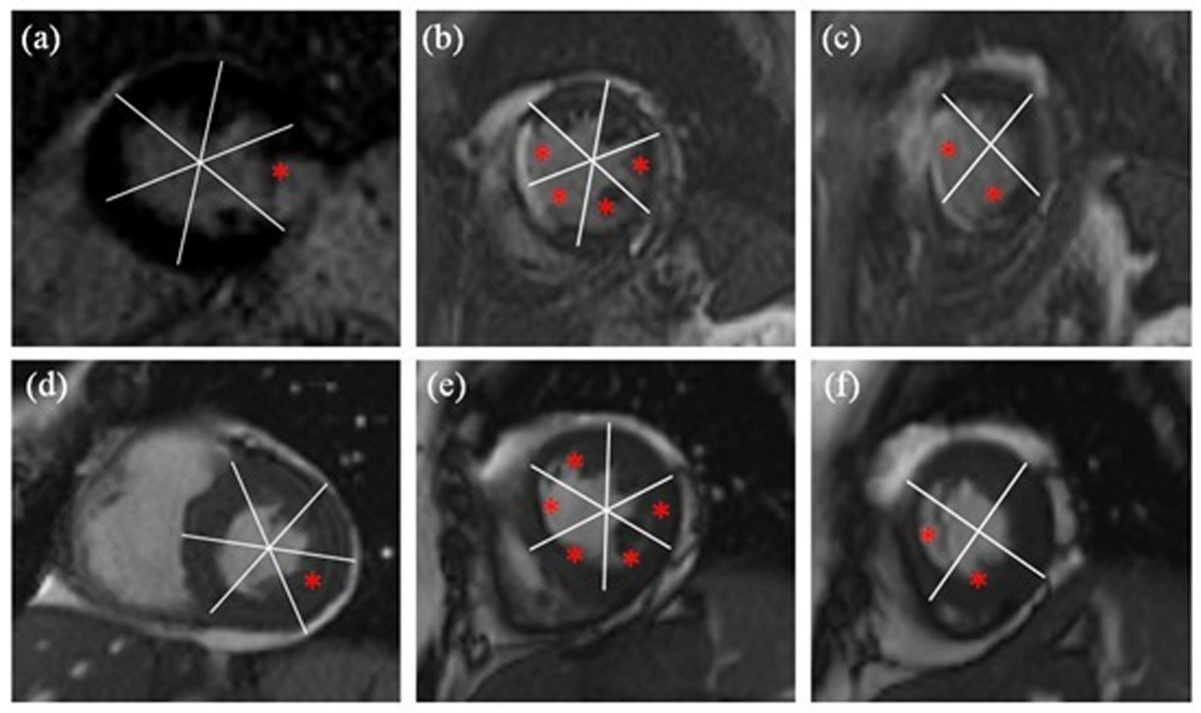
Comparison between infarcted regions using delayed-hyperenhancement MRI (a,b, and c) and regions with motion abnormality detected using the proposed method (d,e, and f).
